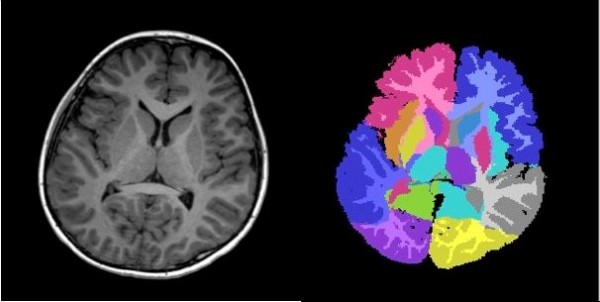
Demonstration of T1-weighted MRI tissue segmentation combined with parcellation of a 9-year-old child’s brain. Axial slice on the left; corresponding combined tissue segmentation/parcellation on the right.
